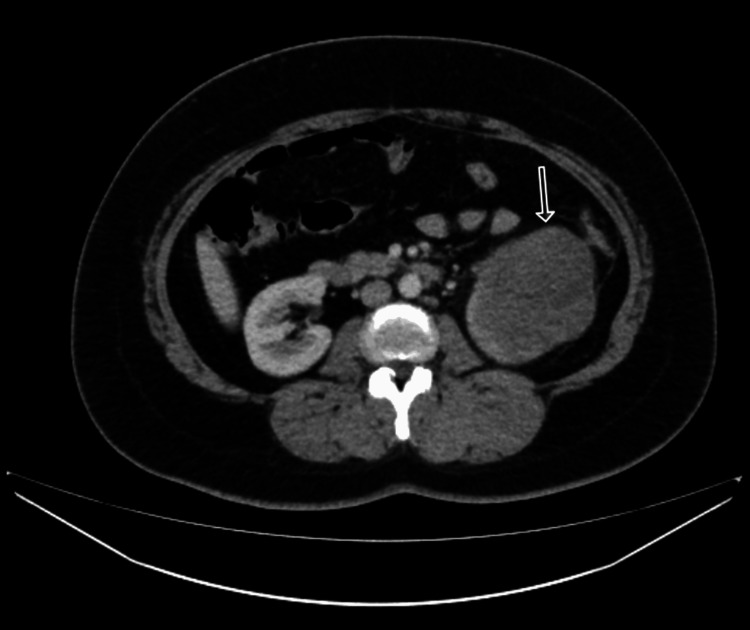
CT abdomen and pelvis with contrast showing an exophytic heterogeneously enhancing mass in the left renal lower pole.
